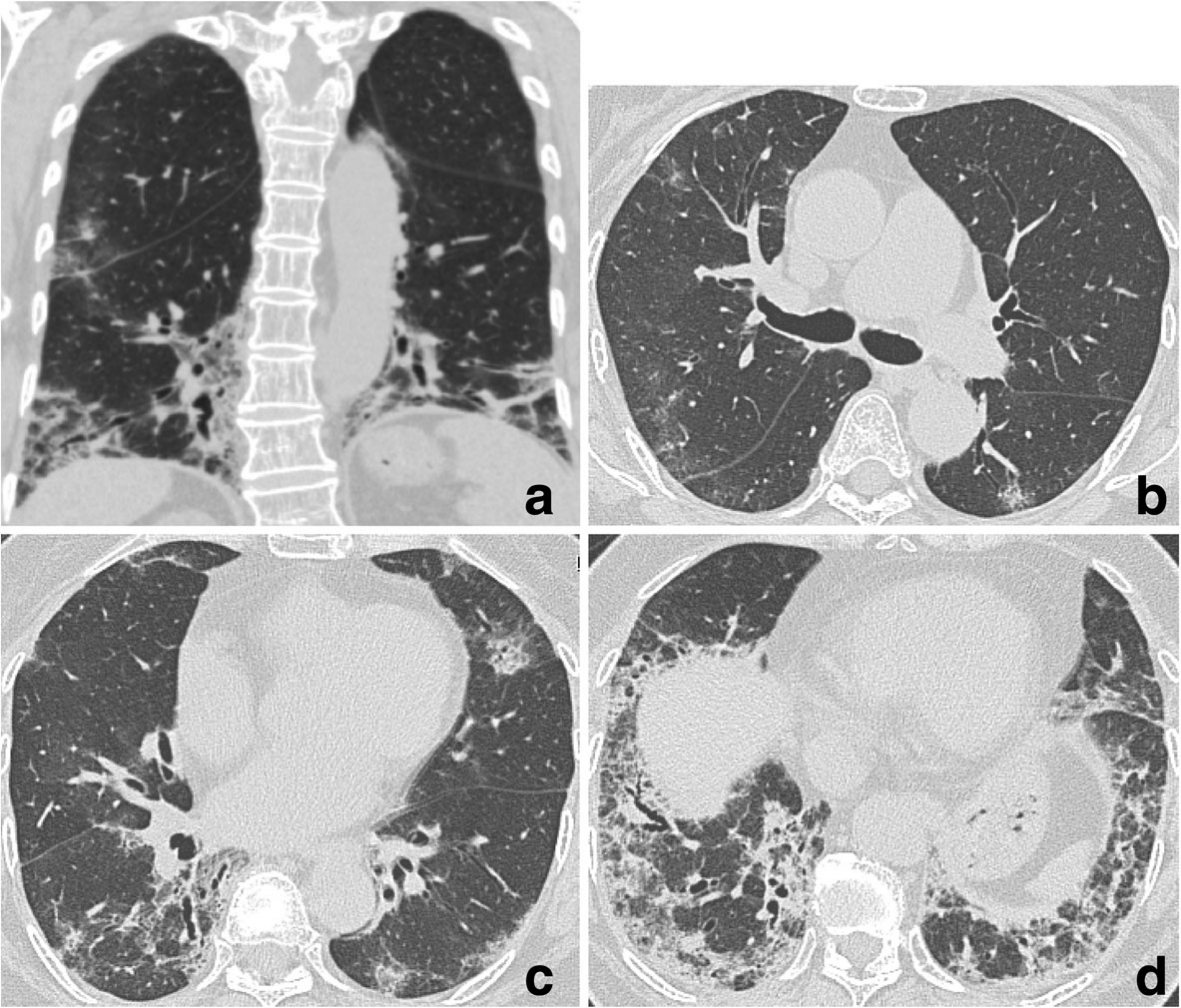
HRCT images of patient #9 (76-years-old female, pathologically cellular NSIP). a The coronal image of the high-resolution computed tomography (HRCT) showed bilateral volume loss and infiltration of lower lung field. b-d Transverse images of the HRCT. Consolidation was observed from middle area to the subpleural area. Traction bronchiectasis was noted inside of consolidation. Reticulation and ground-glass opacity were distributed around consolidation
